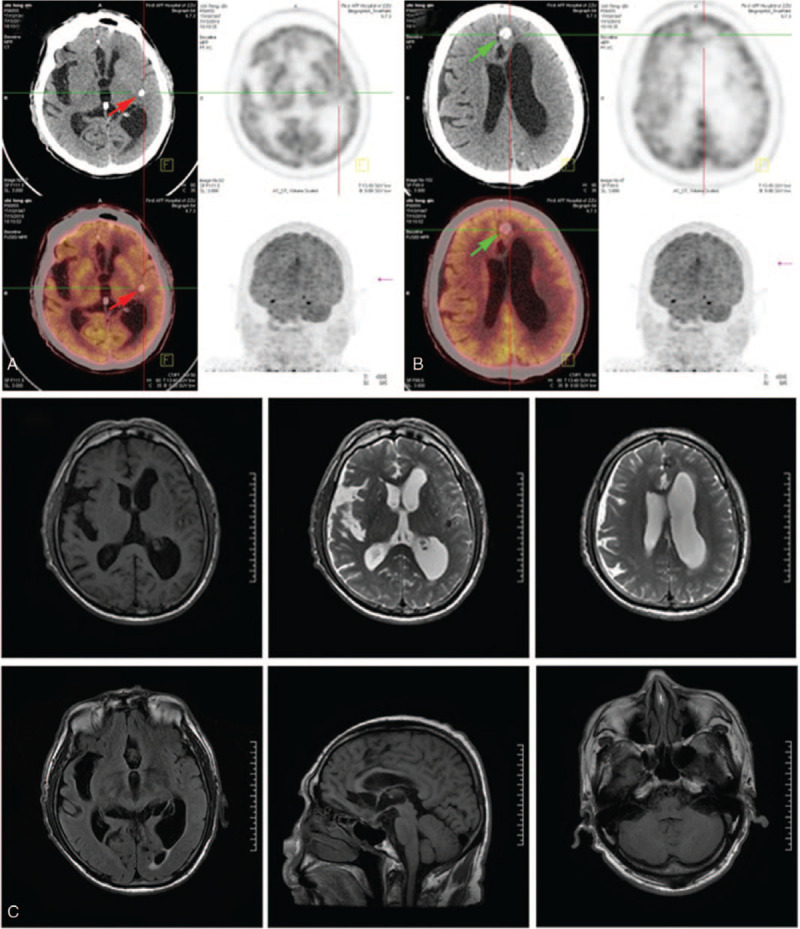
A. Positron emission tomography-computed tomography of head. Red arrow on left indicates a high-density shadow at basal ganglia; B. Green arrow in frontal lobe indicates a high-density shadow, considered calcification from cysticercosis. C. Magnetic resonance imaging (MRI) of head. Anterior longitudinal fissure, suprasellar cisterna, anterior pontine cisterna, multiple abnormal signals in left hippocampus, imaging features of cysticercosis.
